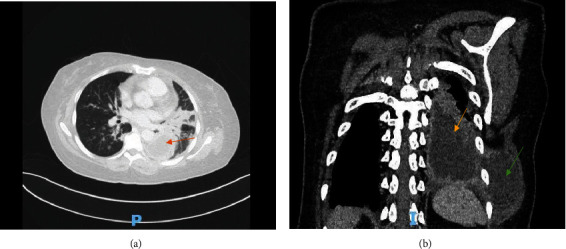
CT scan showing (a) left lower lobe consolidation and atelectatic changes with visualization of pleural collection (orange arrow) and (b) pleural effusion (yellow arrow) in the pleural cavity with extension throughout chest wall (empyema necessitans) (green arrow).
